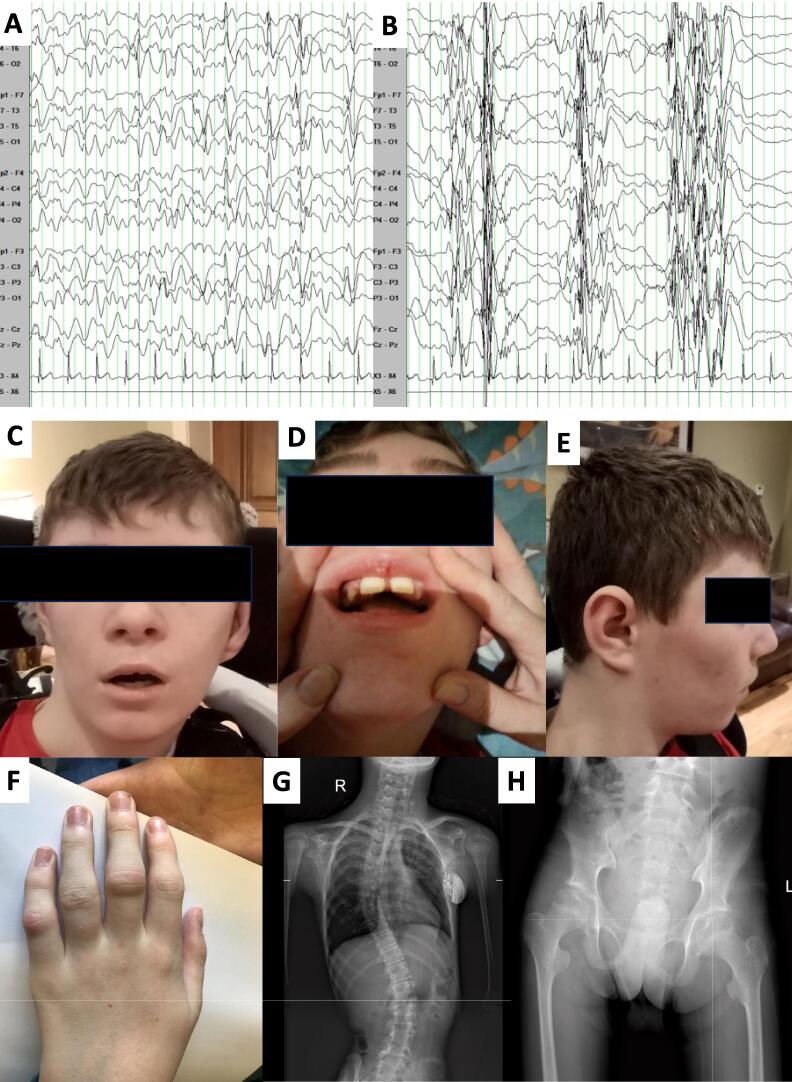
Electroencephalogram (EEG) in awake state showing very slow background and bilateral multifocal slow spike-wave discharges (+/- 1 Hz, <2.5 Hz), (B) in sleep, showing much higher amplitude and impressive bisynchrony with electrodecrements. (C) Triangular facies with anteverted nostrils, bushy eyebrows, long philtrum. (D) Macrodontia of upper central incisors, (E) Brachycephaly. At age 12 years, (F) hand showing clinodactyly of 5th digit, (G) X-ray spine showing convex thoracic scoliosis to the right with lumbar scoliosis to the left (VNS in situ), and generalised osteopenia and (H) narrow underdeveloped pelvis and femora.
